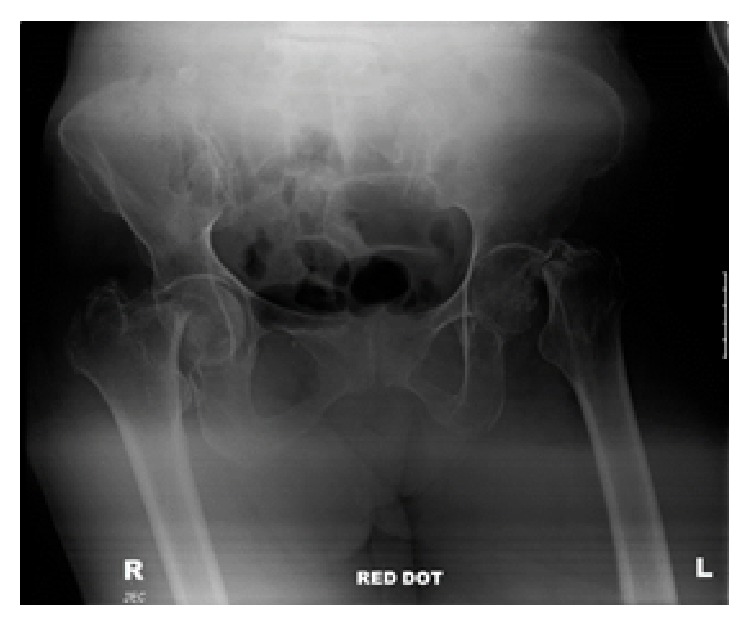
AP X-ray pelvis showing bilateral displaced intracapsular neck of femur fractures.
